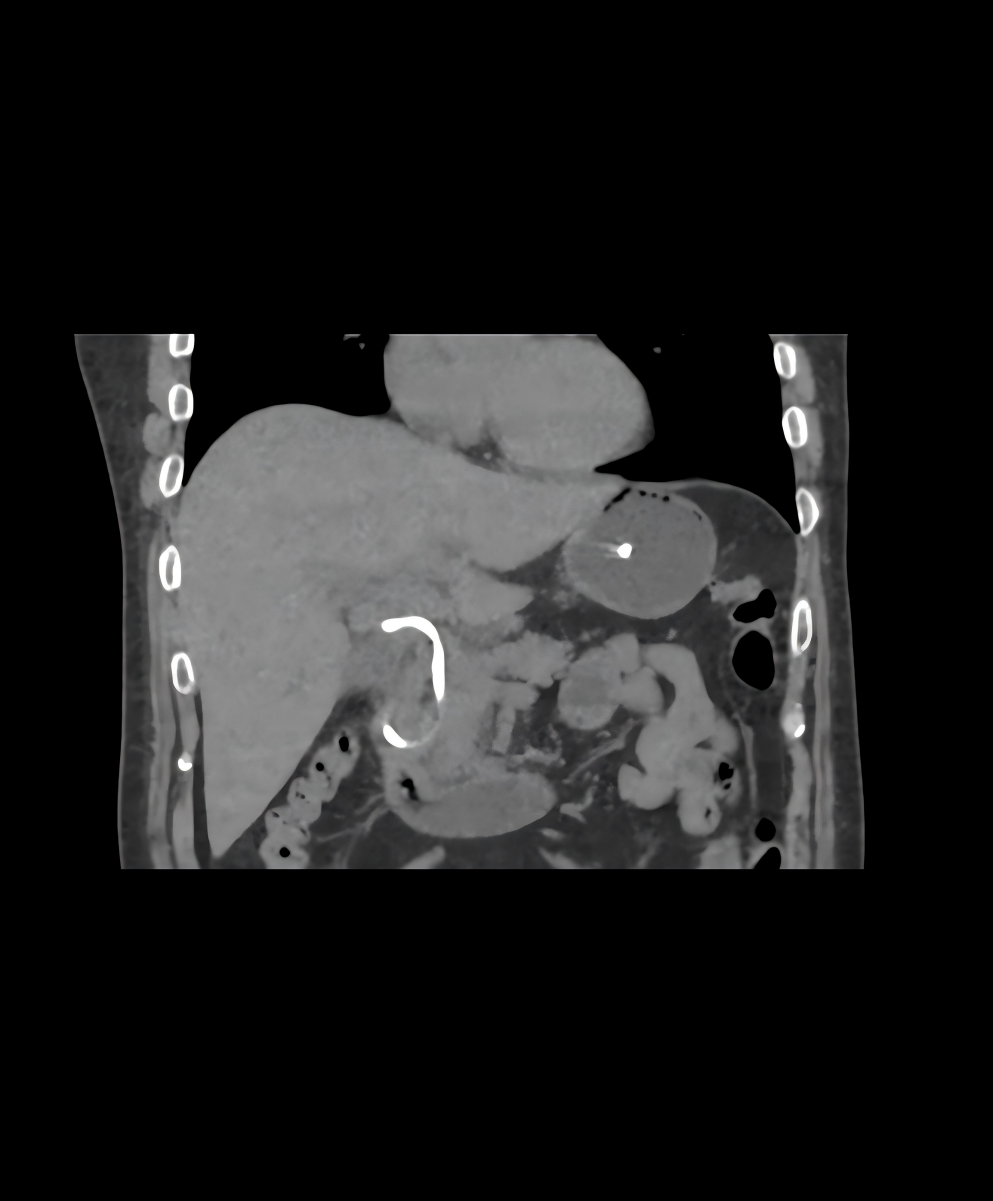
Postoperative follow-up CT scan showed the position of the nasal bile duct was normal, with no displacement or folding.
